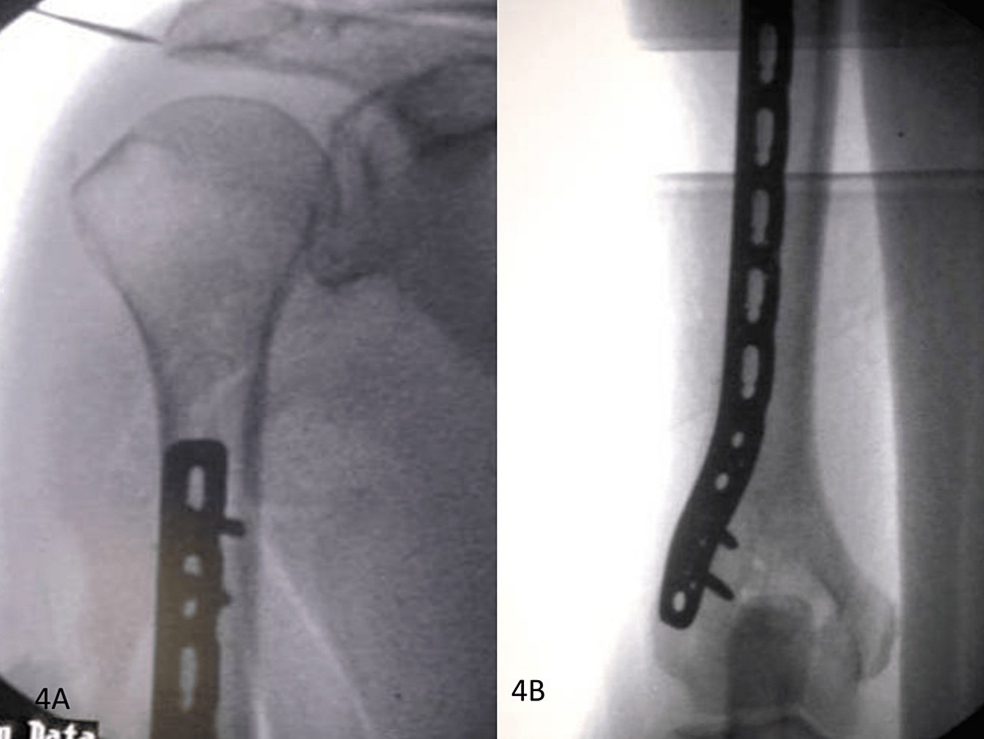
X-ray of the humerus: (A) radiographs (AP view) showing extraarticular distal humeral LCP fixed to the humerus; (B) radiographs (lateral view) showing extraarticular distal humeral LCP fixed to the humerus. AP, anterior-posterior; LCP, locking compression plate
